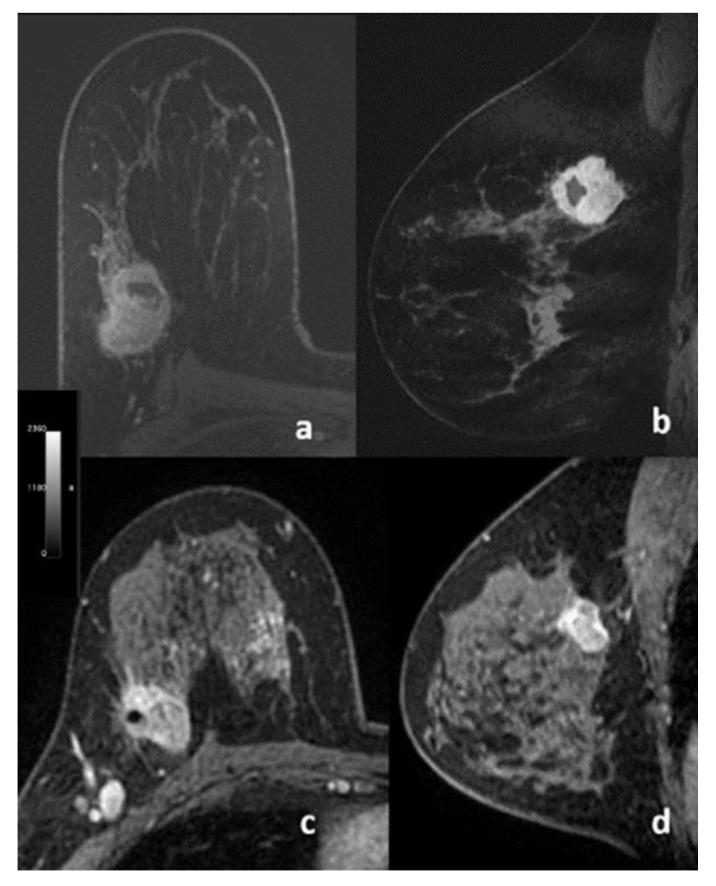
Transverse (a,c) and sagittal (b,d) post-contrast dynamic T1-weighted fat-suppressed MR images of two patients with triple negative breast cancer. (a,b) 33-year-old female with a biopsy-proven, SP142 diagnostic assay PD-L1-positive, poorly differentiated invasive ductal carcinoma. (c,d) 50-year-old female with a biopsy-proven, SP142 diagnostic assay PD-L1-negative, poorly differentiated invasive ductal carcinoma.
